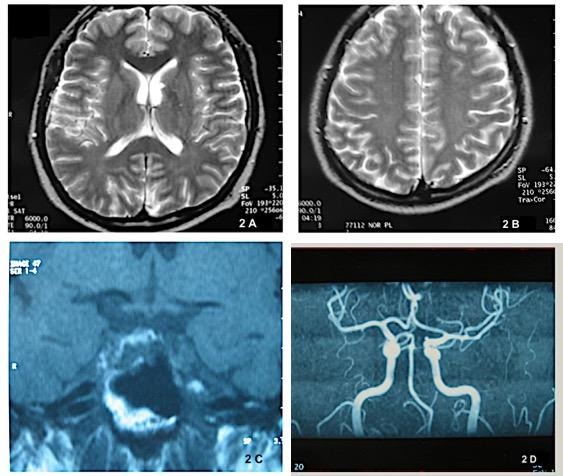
Top left (A): T2 W MRI of the brain shows a hyperintensity consisted with lacunar infarct in the head of caudate nucleus and adjacent to the ventricle. Top right (B): T2 W MRI of brain shows three hyperintense areas consistent with lacunar infarct of white matter in the level of centrum semiovale. Bottom left (C): T1 W coronal plane MRI of hypophysis demonstrates an isointense mass in the right half side of the hypophysis lying into sphenoid sinus, displaying osseous destruction and does not show an enhancement of contrast agent. Bottom right (C): 3D-TOF MRI Angiography demonstrates absence of P1 branch of the left posterior cerebral artery
